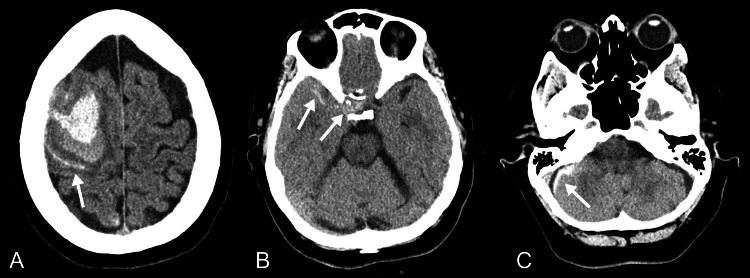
Initial unenhanced head CT images. (A) Axial images demonstrate a right frontal intraparenchymal hematoma with adjacent convexity SAH (arrow).
